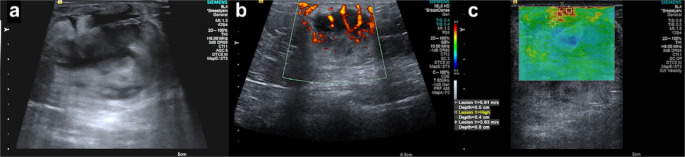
Breast plasmacytoma in a 55-year-old woman. (a) B-mode ultrasound image demonstrates a lobulated-shape mass with indistinct margins, heterogeneous echotexture, parallel orientation, and a prominent echogenic rind. Marked posterior acoustic enhancement is also observed. (b) Power Doppler ultrasound image reveals vascularity within the lesion. (c) Shear-wave elastography image demonstrates high tissue stiffness
